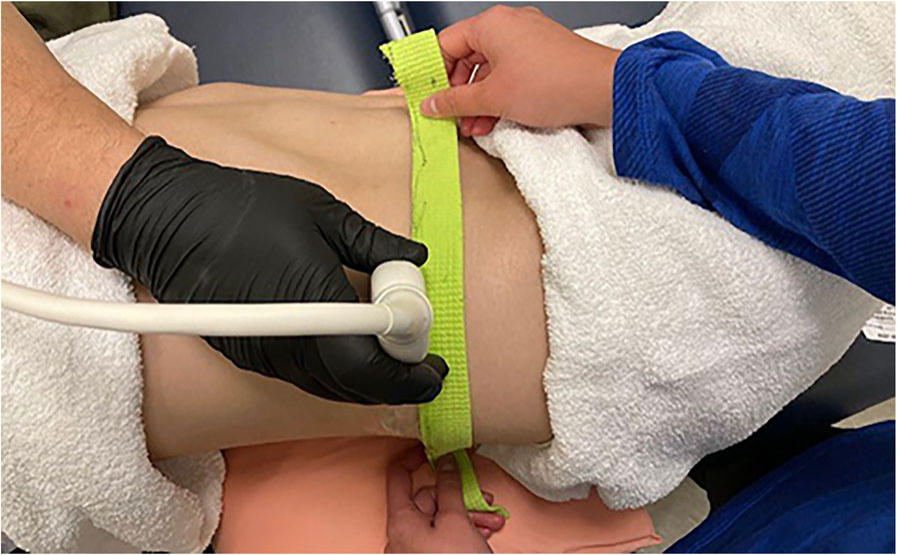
Extended field of view ultrasound imaging of the transversus abdominis muscle in side-lying with guide strip to maintain appropriate spinal level
